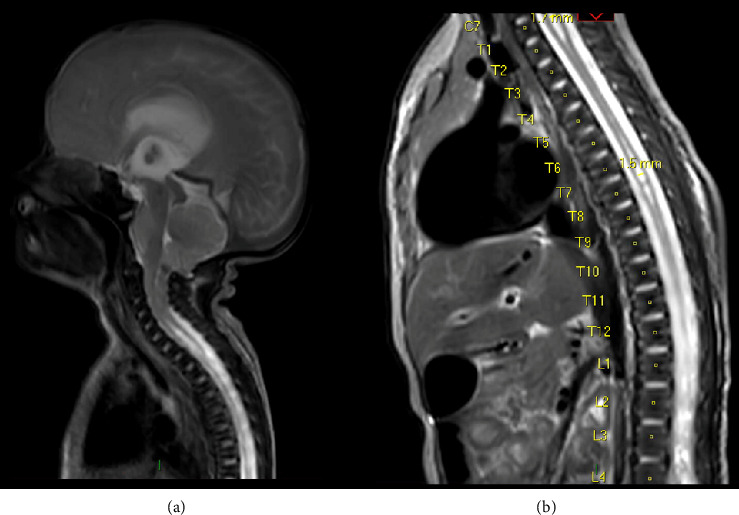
Preoperative T2 weighted sagittal MRI scan demonstrating (a) Chiari II malformation with cervical syringomyelia; (b) cervical and thoracic syringomyelia (from patient notes).
